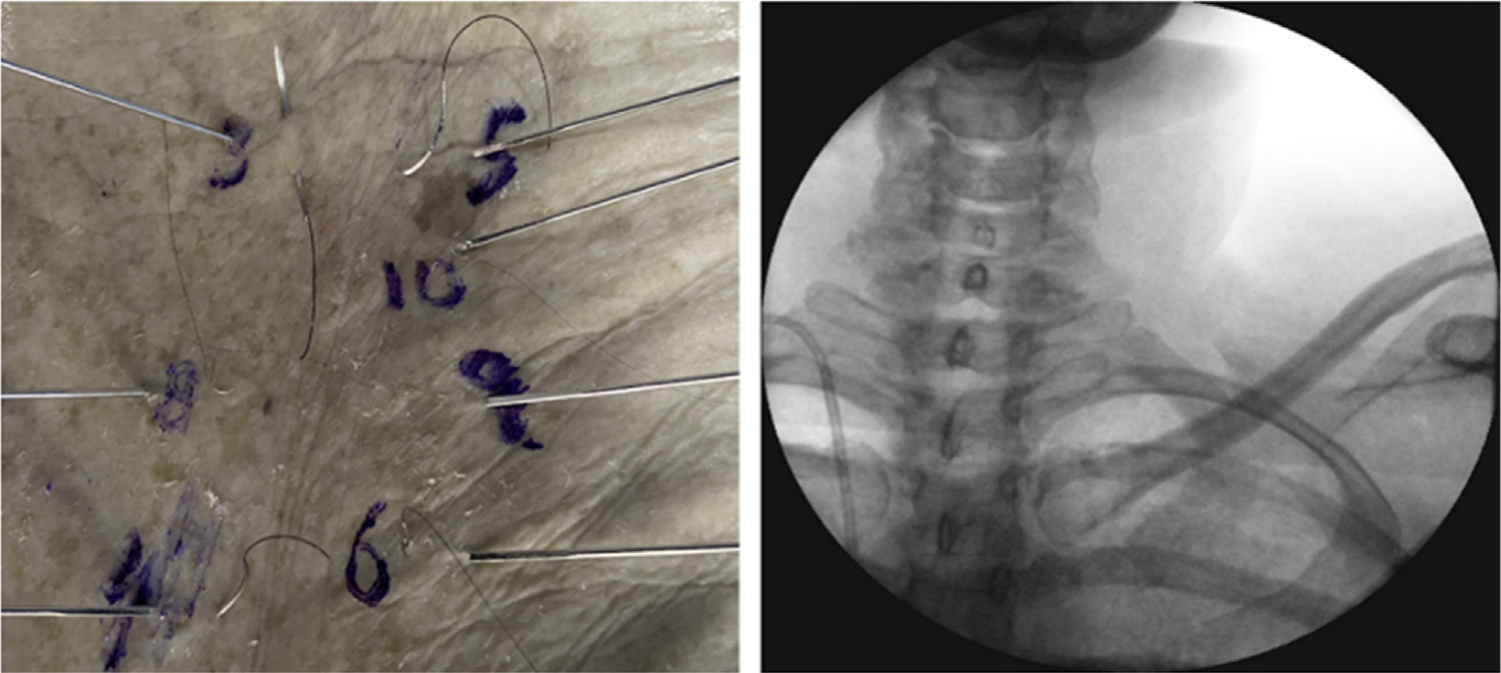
Second intercostal space images/radiograph for needle identification.
